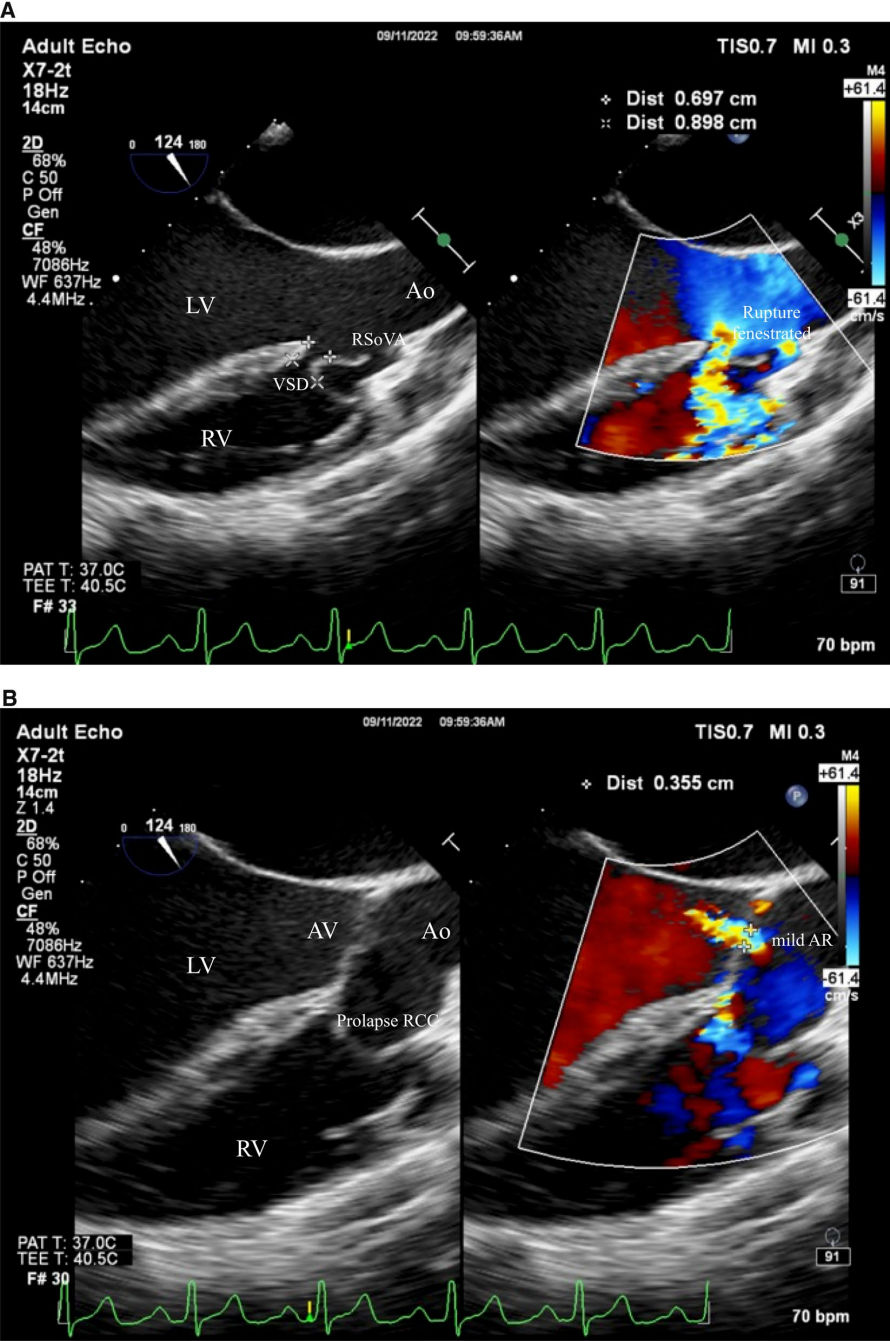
A Transoesophageal echocardiography showed subaortic VSD left-to-right shunt. B Mild aortic regurgitation due to prolapse of RCC. Ao = aorta; RV = right ventricle; LV = left ventricle; RSoVA = ruptured sinus of Valsalva aneurysm; VSD = Ventricular septal defect
